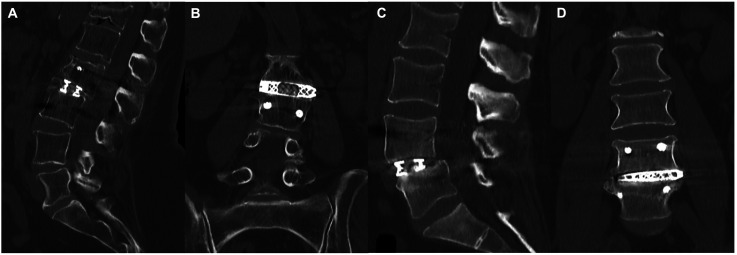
CT scans at 1-year postoperatively. A-B: A patient who underwent single-level LLIF L2/3 with a Grade I fusion at 1-year postoperatively. C-D: A patient who underwent a single-level LLIF L4/5 with a Grade II fusion (partial fusion) at 1-year postoperatively.
